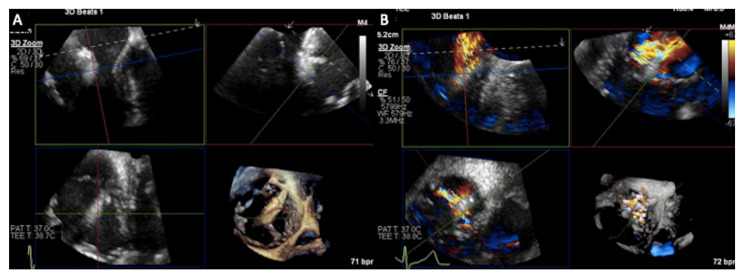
Intraprocedural transesophageal echocardiography. Multiplanar views and derived 3D reconstructions (A), and with color Doppler in (B) are crucial to help correct clip positioning.
